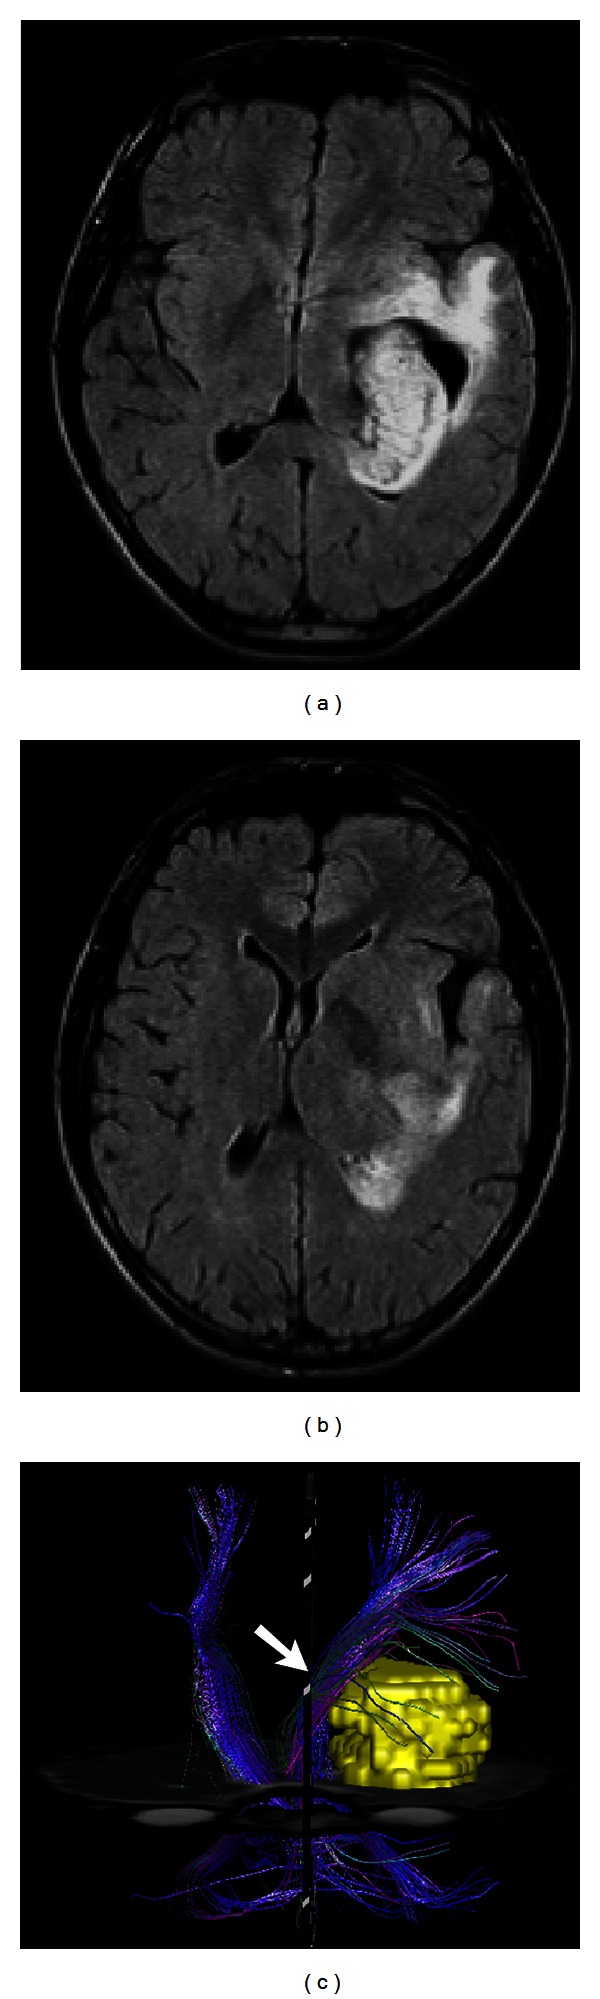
((a), (b)) FLAIR images depict a diffuse and infiltrative oligodendroglioma, WHO grade III, which deviates the corticospinal tract (arrow in (c)) medially, as demonstrated on DTI tractography (c).
